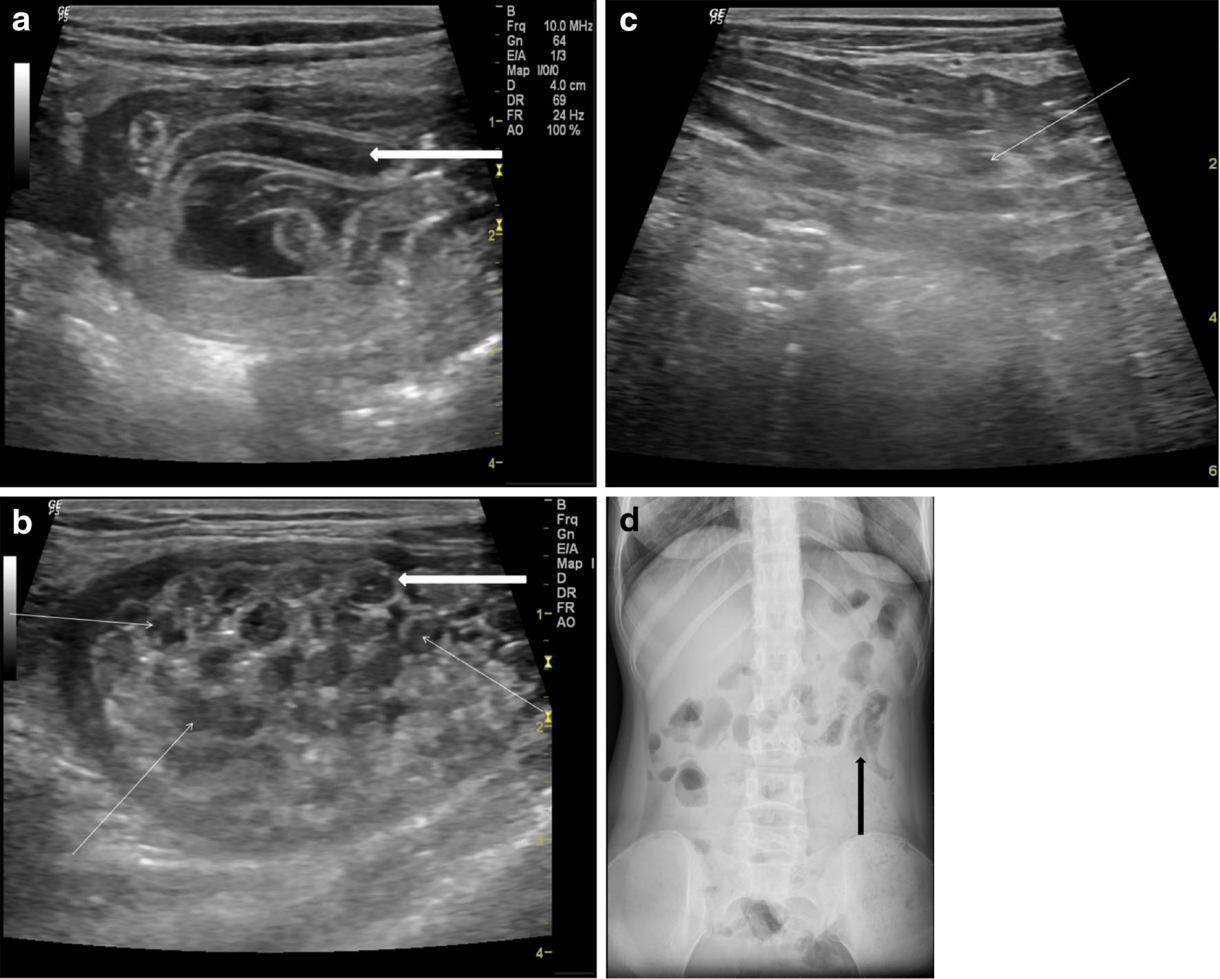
A 25-year-old female with acute abdomen and clinical signs of intestinal obstruction. (a) High resolution ultrasound using linear array transducer showing intestinal ascariasis, note distended fluid filled alimentary canal of the ascaris (*thick white arrow*), the “inner tube sign”, (b) same patient showing multiple ring like shadows (*thin white arrows*), some with target appearance (*thick white arrow*) on transverse section of the intestinal lumen indicating bag of worms, (c) “Bag of worms” in a long section (*thin white arrow*) and (d) PA erect X-ray abdomen of this patient showing tubular soft densities suggestive of ascariasis (*thick black arrow*)
